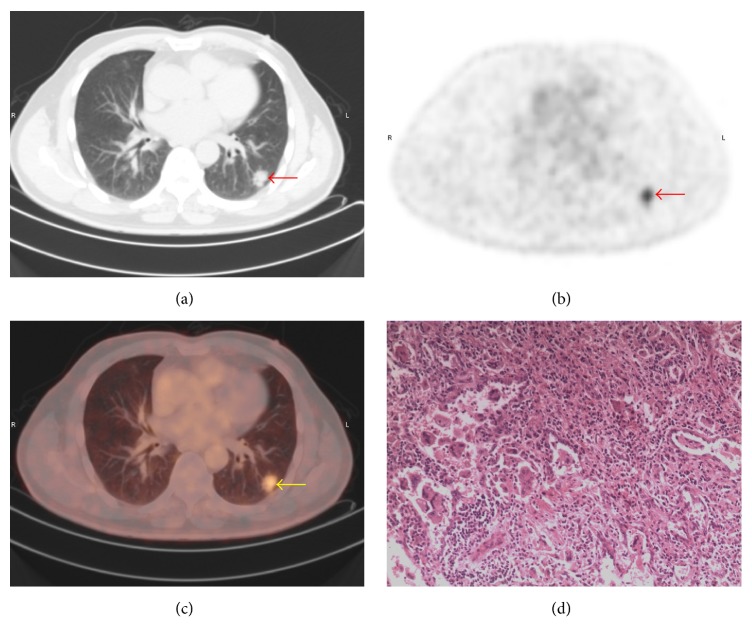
A false-positive PET/CT finding in a 55-year-old male patient. (a) On CT image, the nodule in the left lower lobe with lobulation, spiculation, and vacuole sign is observed (arrow); the maximum diameter of nodule is 18 mm. (b) On PET and (c) on PET/CT image, increased ^18^F-FDG uptake is detected; SUV_max_ = 2.2. (d) Pathologic examination suggests cryptococcal infection. The *y* value calculated by our model is 0.91. The result of our model is consistent with PET/CT.
